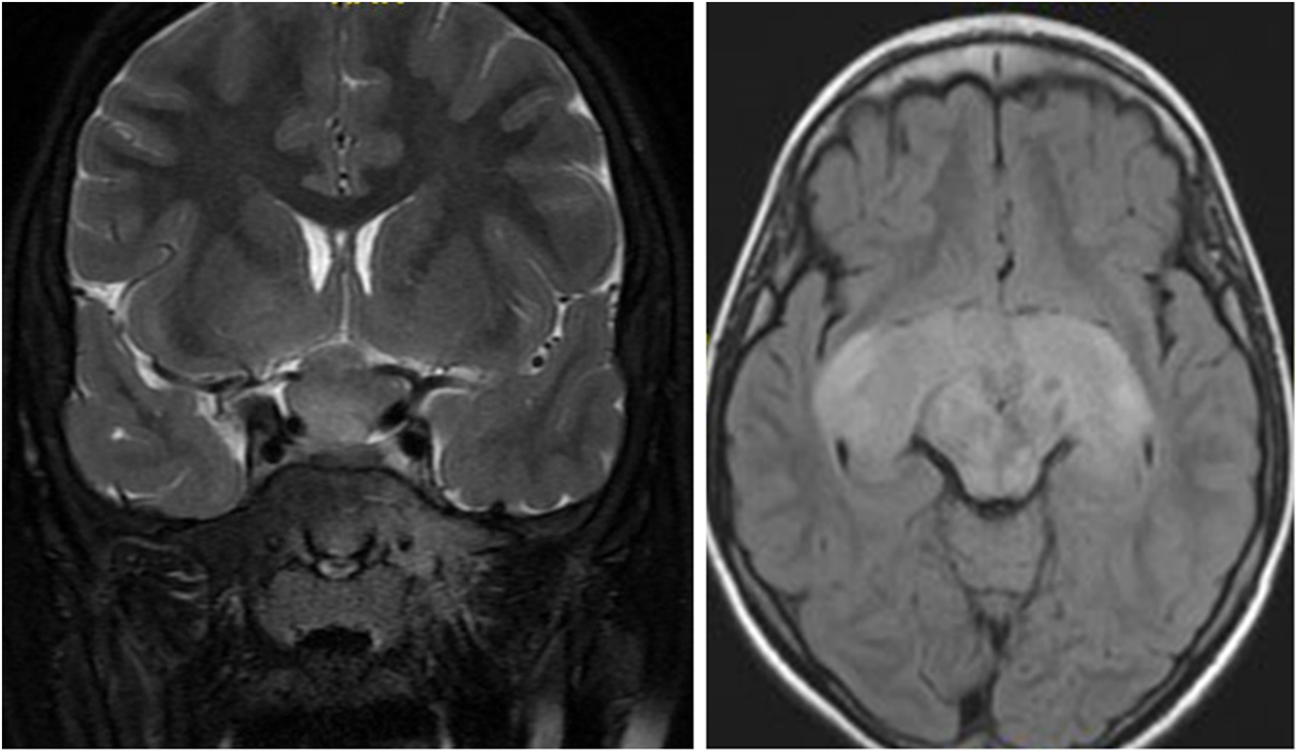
Optic pathway glioma (OPG) in neurofibromatosis type I (NF1): coronal T2-weighted MRI and axial FLAIR MRI of a 9-year-old boy with NF1. MRI shows a typical OPG, involving the optic nerves, chiasm, and optic tracts. Typical NF changes are seen in the mid-brain. The child underwent treatment with vincristine and carboplatin, followed by vinblastine. Over the years, the tumor reduced in size; however, vision continued to deteriorate
